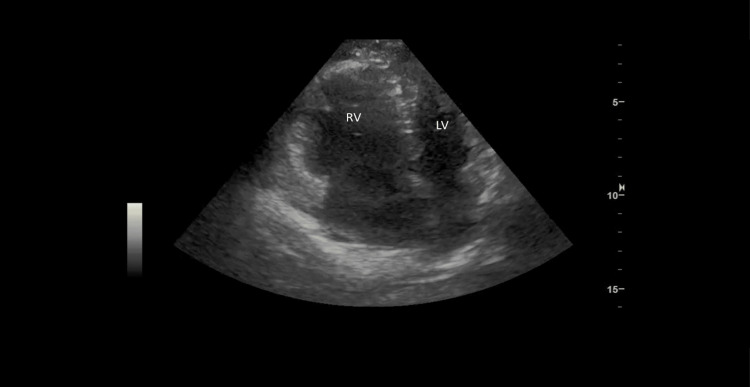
Apical four-chamber view of the heart showing massively dilated right ventricle (RV) as compared to the left ventricle (LV)
